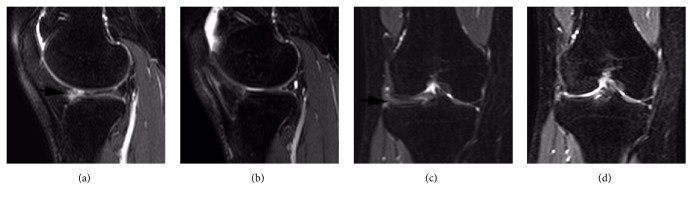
(a), (c) Horizontal tear of the anterior horn of the lateral meniscus was observed in a sagittal and coronal MRI in a 19-year-old college soccer player (black arrow). (b), (d) Sagittal and coronal MRIs one year after the operation revealed the resected inferior leaf of the lateral meniscus anterior horn.
